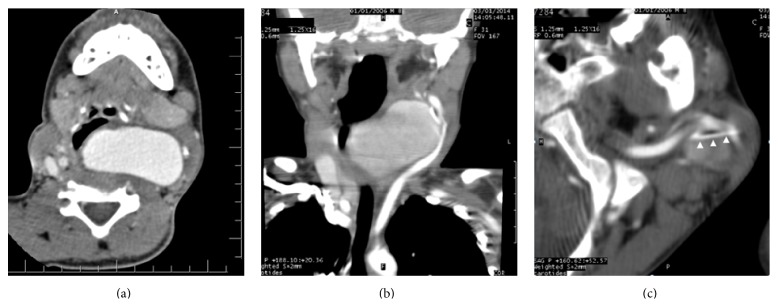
Axial (a) and coronal reformatted (b) contrast-enhanced CT scan images of the neck showing a saccular dilatation of the left common carotid artery with mass effect on the trachea and adjacent vascular structures. Axial bone window image (c) along the upper portion of the aneurysm shows a linear hyperdensity denoted with arrow-heads (later confirmed to be a fish bone).
